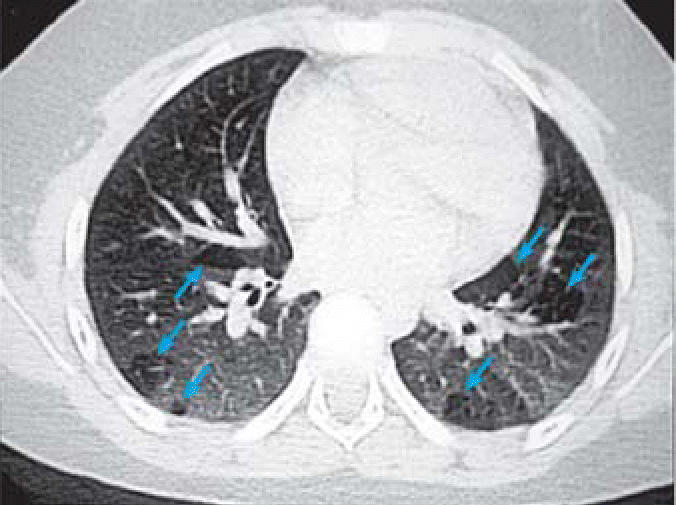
High-resolution expiratory CT of a 9-year-old boy demonstrating air trapping at the level of the secondary pulmonary lobule (arrows).
